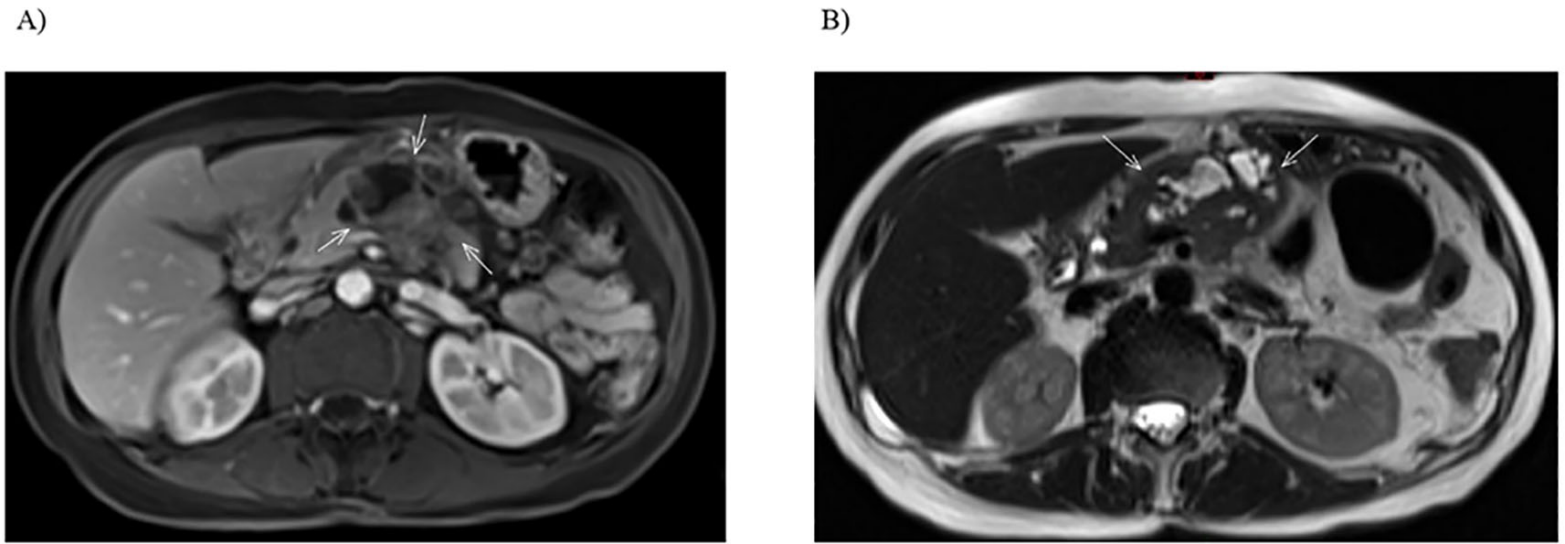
Imaging of the pancreatic lesion before biopsy. (A) Axial T1-weighted post-contrast MRI shows a solid-cystic tumor in the pancreatic body, with invasion of the superior mesenteric vein and anterior extension towards the abdominal wall. (B) Axial T2-weighted MRI highlights the cystic component of the lesion and demonstrates infiltration into the jejunal loop.
